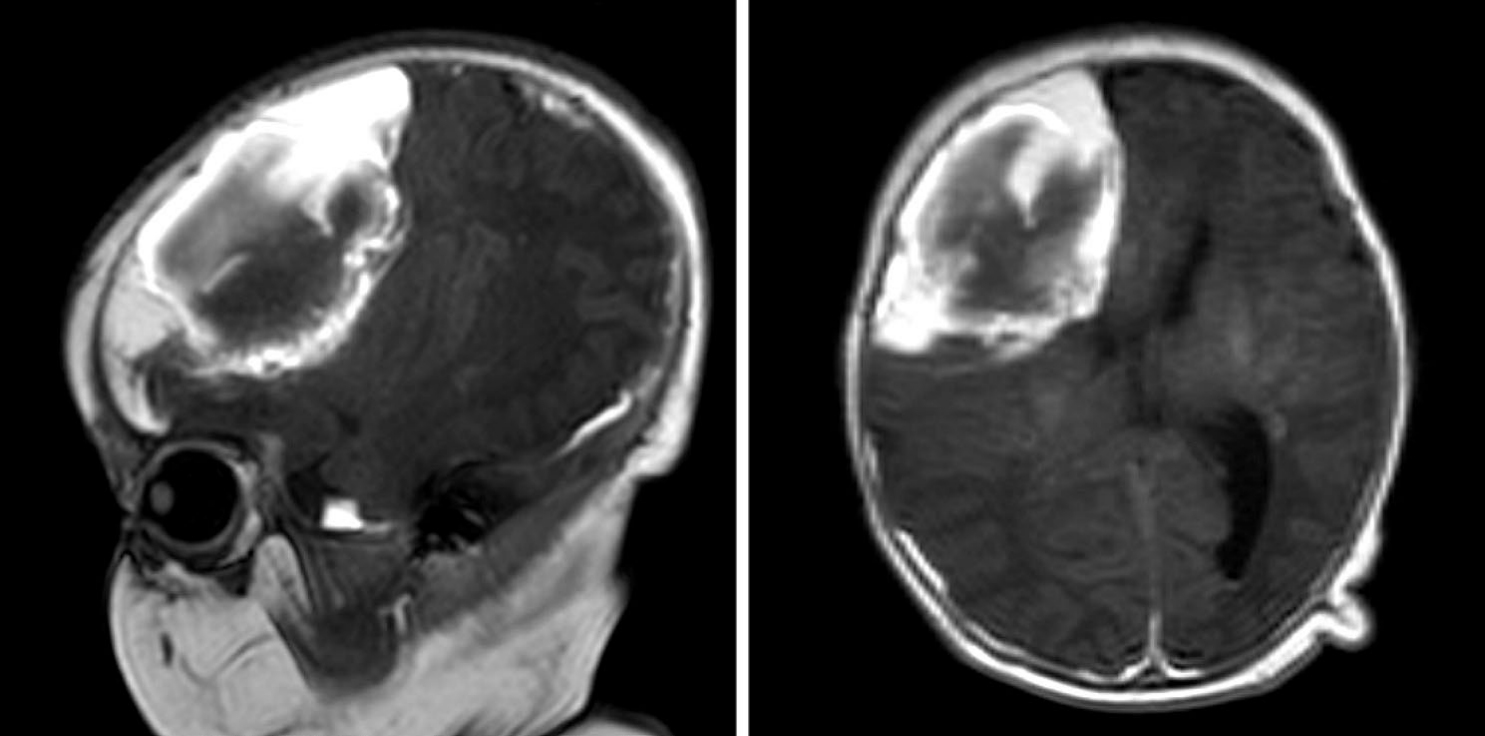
Utility of MRI. Sagittal (Left) and axial (Right) T1-weighted MR images showing a large intraparenchymal hemorrhage
